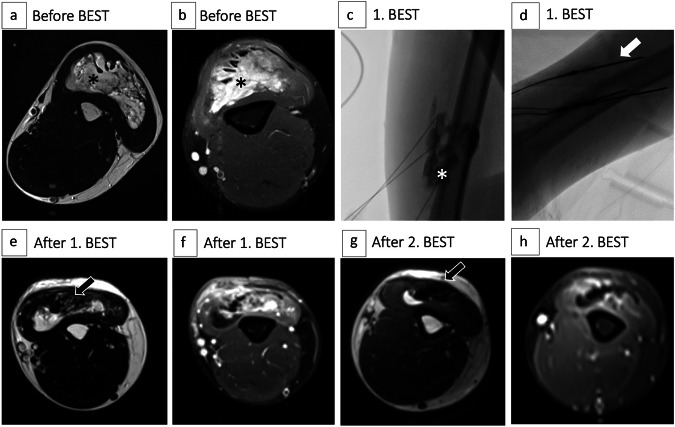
27-year-old male patient with intramuscular venous malformation (VM) of the upper extremity undergoing two subsequent bleomycin electrosclerotherapies (BESTs). a, b T2-weighted (T2w) and fat-suppressed (fs) T1-weighted (T1w) axial magnetic resonance (MR) images prior to treatment with BEST showing the extent of a symptomatic VM (asterisks) predominantly located in the triceps brachii muscle. c, d Digital subtraction angiography (DSA) images during first BEST session. The asterisk shows the filling of the dysplastic venous structures of the lesion after KM insertion. The arrow mark shows the parallelly inserted needle electrodes during therapy. e, f T2w and fs T1w axial MR images three months after the first BEST session revealing significant regredience of the vascularized parts of the VM (arrow) with accompanying improvement in the patient’s pain symptoms. g, h T2w and fs T1w axial MR images three months after the second BEST revealing near-complete resolution of the malformation. The arrow mark shows the volume-reduced lesion
